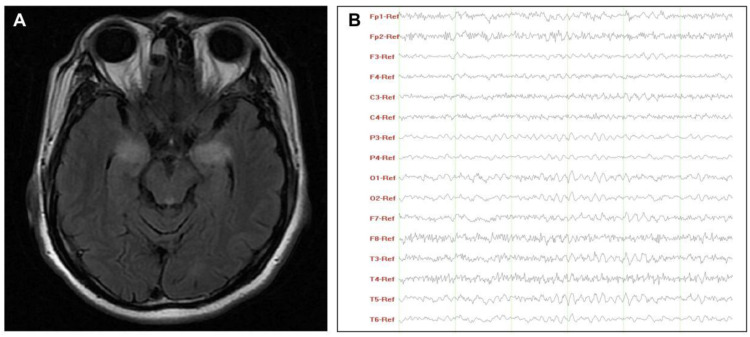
Characteristics of MRI (A) and EEG (B) of patient No.6. (A) T2-weighted images showed hyperintensity lesions on the bilateral mesial temporal lobe; (B) EEG revealed a widely diffuse distribution of theta waves with some low-amplitude fast activity. Abbreviations: EEG, electroencephalograms; MRI, magnetic resonance imaging.
